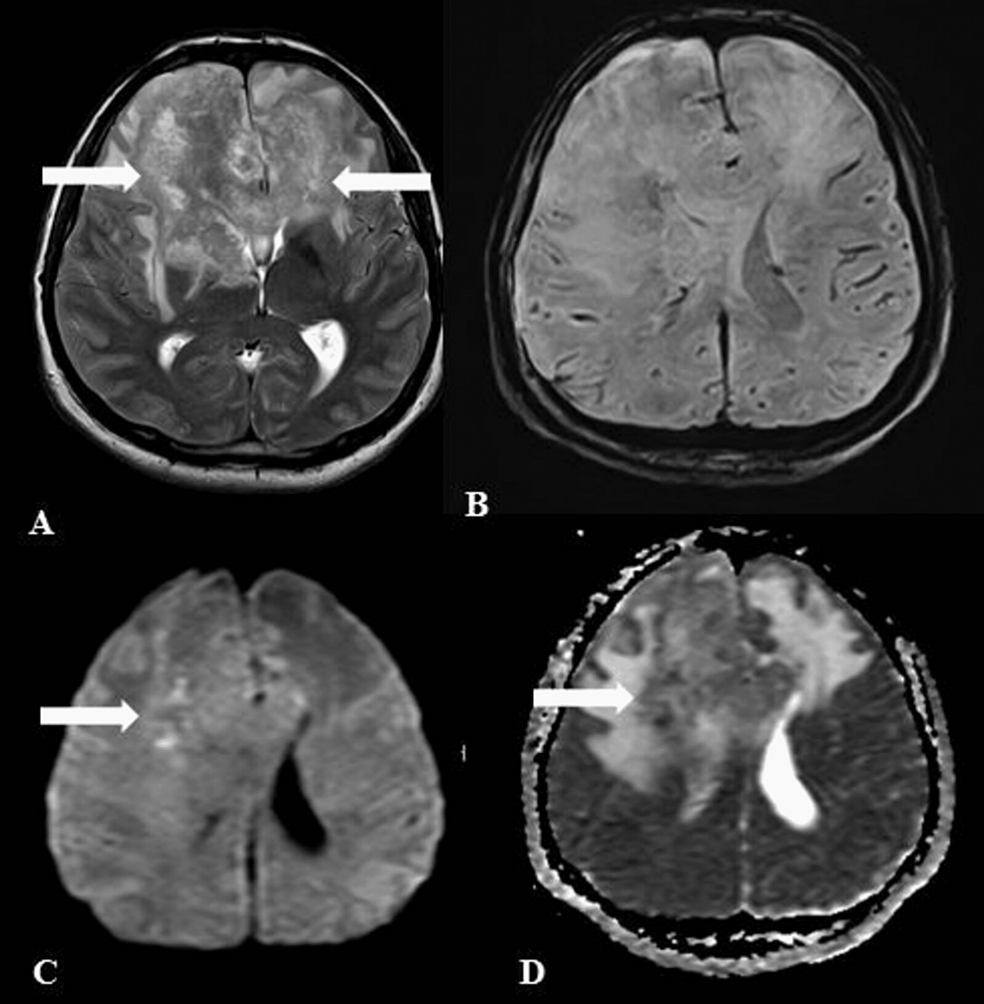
Magnetic resonance images of the brain. Axial T2-weighted sequence (A) showing an infiltrative heterogeneously hypointense lesion in the anterior cranial fossa and frontal lobes, with surrounding perilesional edema (arrows). No susceptibility seen on SWI sequences (B). Patchy foci of diffusion restriction (arrows) noted within it on DWI and ADC maps (C&D). SWI: susceptibility-weighted imaging; DWI: diffusion-weighted imaging; ADC: apparent diffusion coefficient
